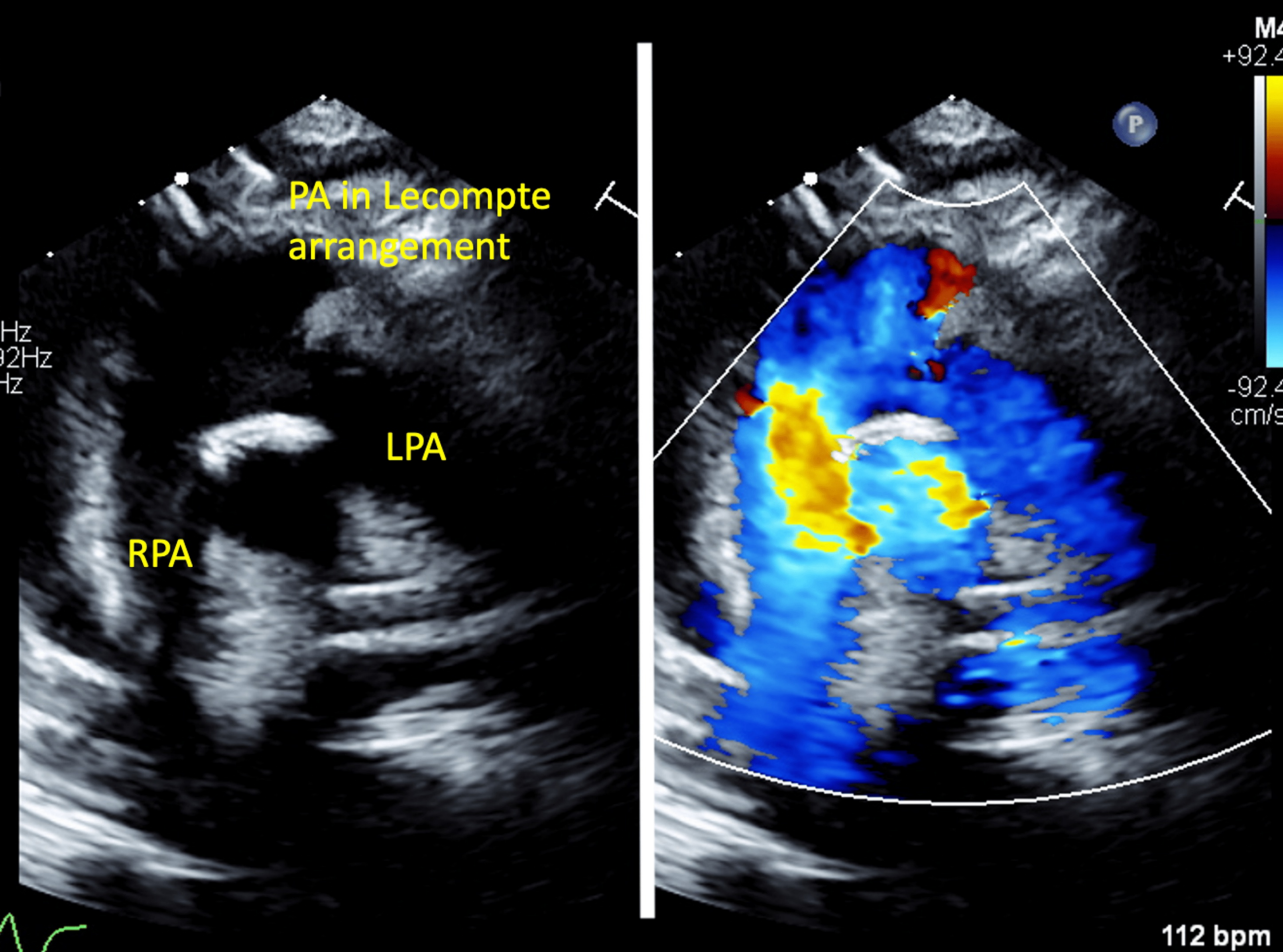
Postoperative echocardiogram (modified suprasternal view) showing the patent right ventricular outflow tract LPA, left pulmonary artery; PA, pulmonary artery; RPA, right pulmonary artery
